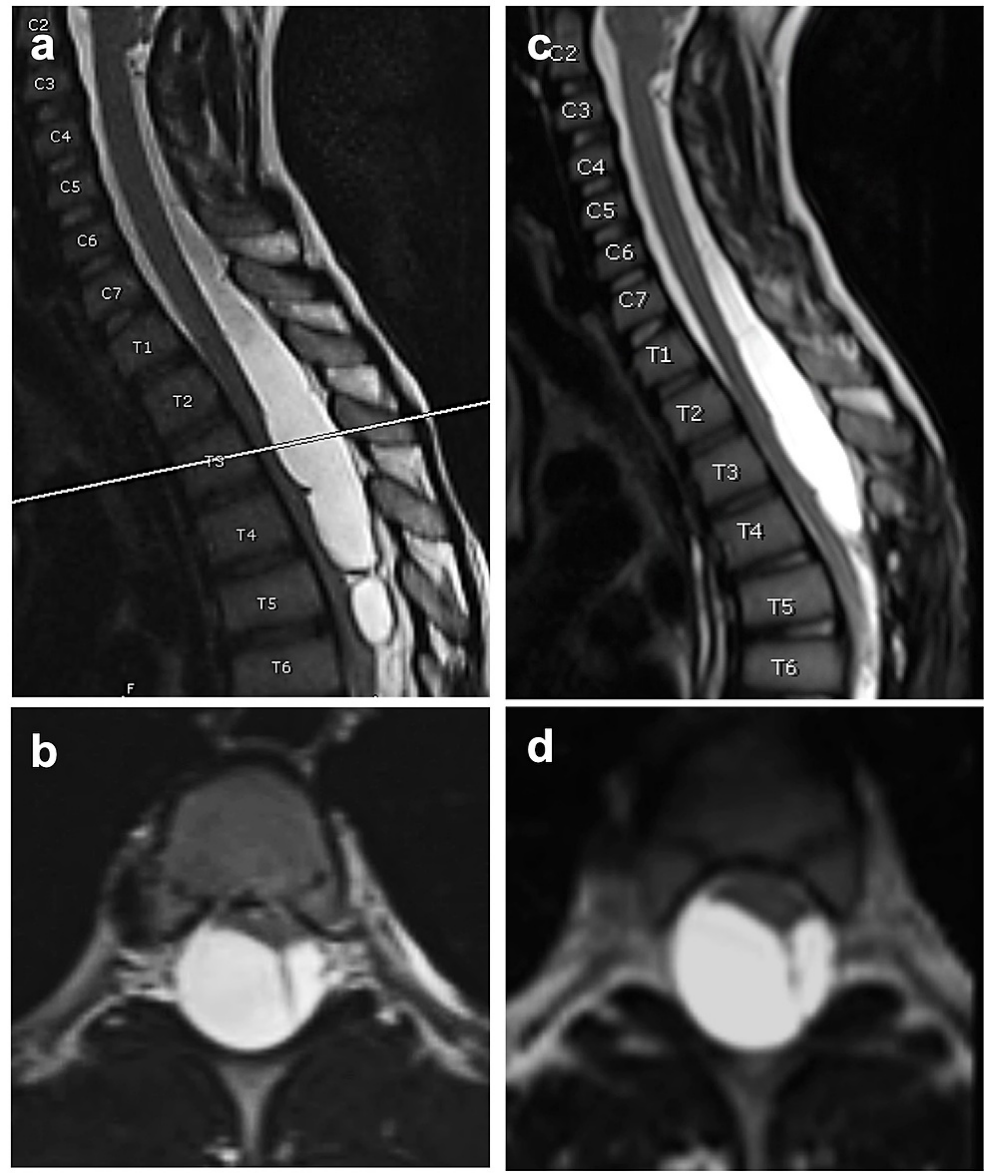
Preoperative and postoperative MRI. (a and b) Preoperative MRI demonstrating arachnoid cyst with significant mass effect on the spinal cord. (c and d) Three-month postoperative MRI demonstrating unchanged cyst and mass effect on the spinal cord.
